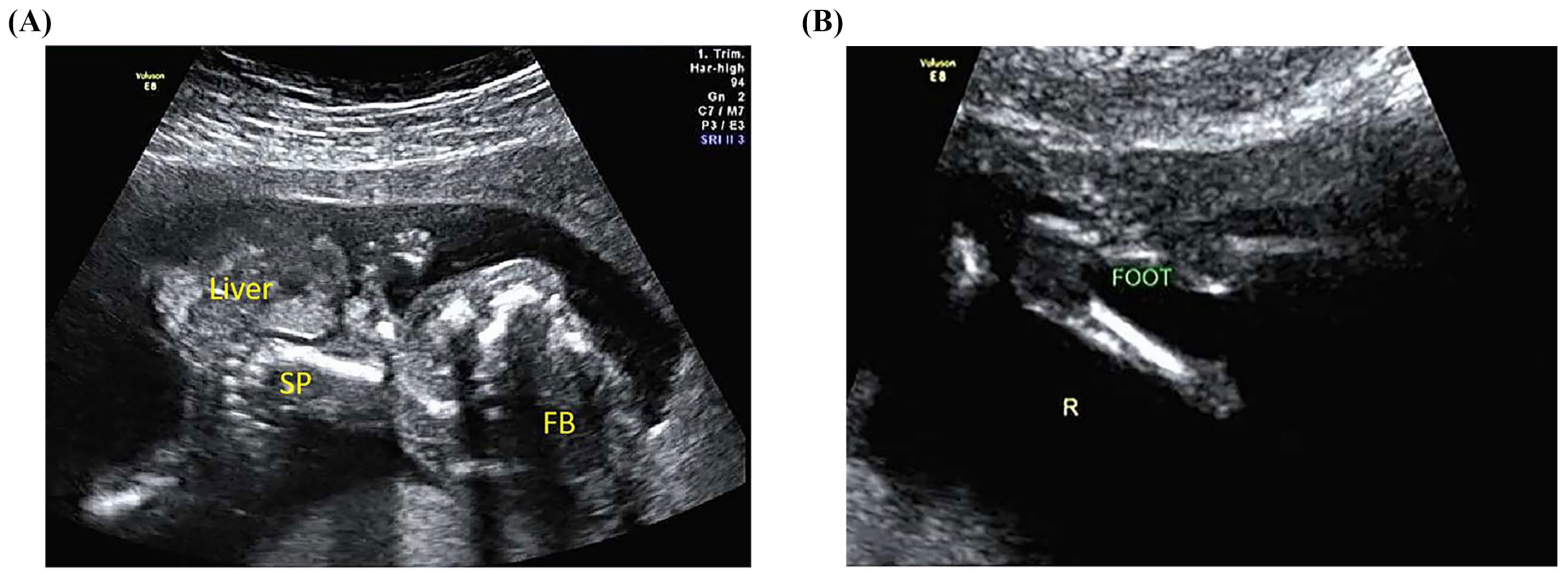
Ultrasound images of a fetus with limb–body wall complex in a 36-year-old woman. (A) The grayscale ultrasound shows the absence of the cranial bone halo, severe kyphoscoliosis of approximately 60 degrees, and herniation of abdominal viscera with an inner echo similar to the liver. SP: spine, FB: fetal brain. (B) Normal right foot morphology is not clearly visible on grayscale ultrasound. R: right.
